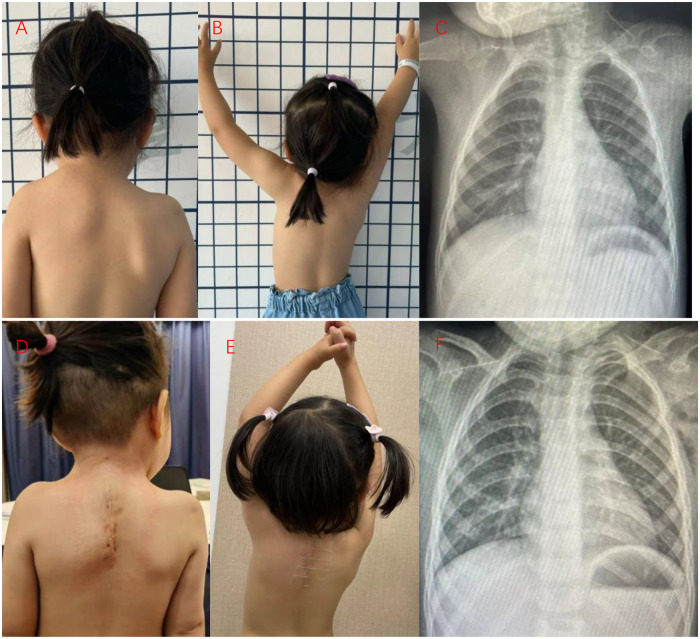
Pre- and postoperative comparison of a typical case associated with klippel-feil syndrome (3-year-old female, cavendish grade III). (A) Preoperative posterior view clearly demonstrating elevated scapulae and short neck deformity. (B) Preoperative maximum active shoulder abduction photograph showing functional limitation. (C) Preoperative anteroposterior (AP) radiograph revealing high scapulae. (D) Postoperative dorsal view showing markedly improved scapular position with bilateral symmetry and an aesthetically pleasing short scar. (E) Postoperative maximum active shoulder abduction demonstrating near-normal range of motion. (F) Postoperative AP radiograph confirming scapular descent to the normal level.
